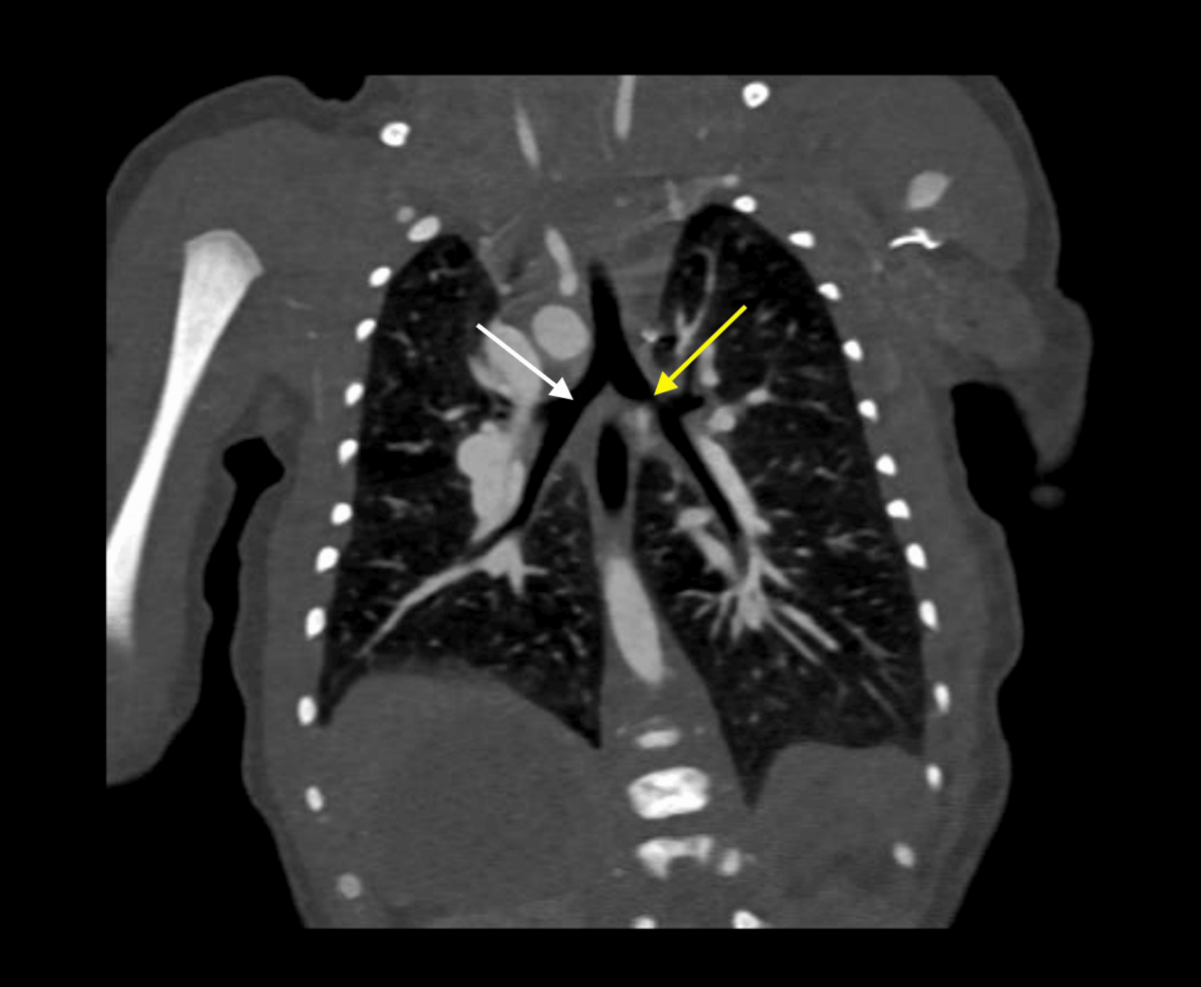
Coronal contrast-enhanced computed tomography image demonstrating bilateral right-sided tracheobronchial branching The yellow arrow identifies the right main bronchus with typical short and vertical right-sided morphology. The white arrow indicates the left main bronchus, which mirrors the right in its configuration, consistent with bilateral right-sided bronchial anatomy.
